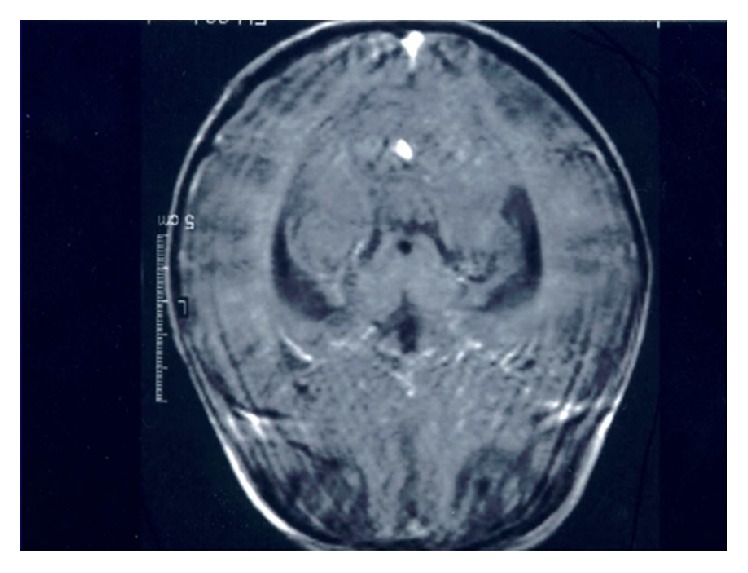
Basilar enhancement on cranial MRI.
